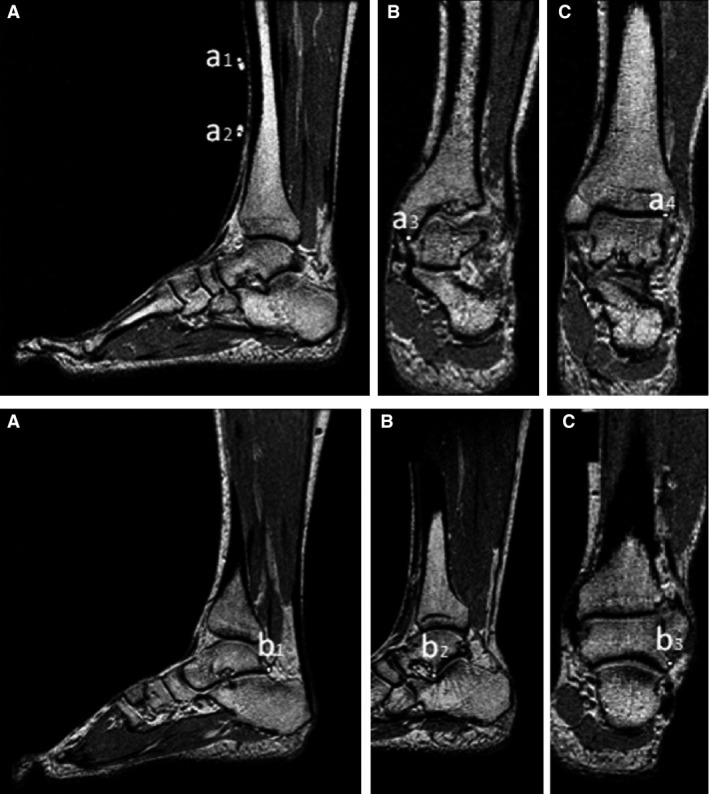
MR images of the right foot for determining the bony landmarks of the tibia (a1–a4) and the talus (b1–b3) used for defining the local coordinate system embedded to each bone. The 3D coordinates of the bony landmarks were obtained using a computer‐aided diagnosis system (PLUTO, Nagoya University). This figure is cited from Figures 2 and 3 of “In vivo determination of the Achilles tendon moment arm in three‐dimensions (Hashizume et al. 2012)” in the *Journal of Biomechanics* 45 (2).
